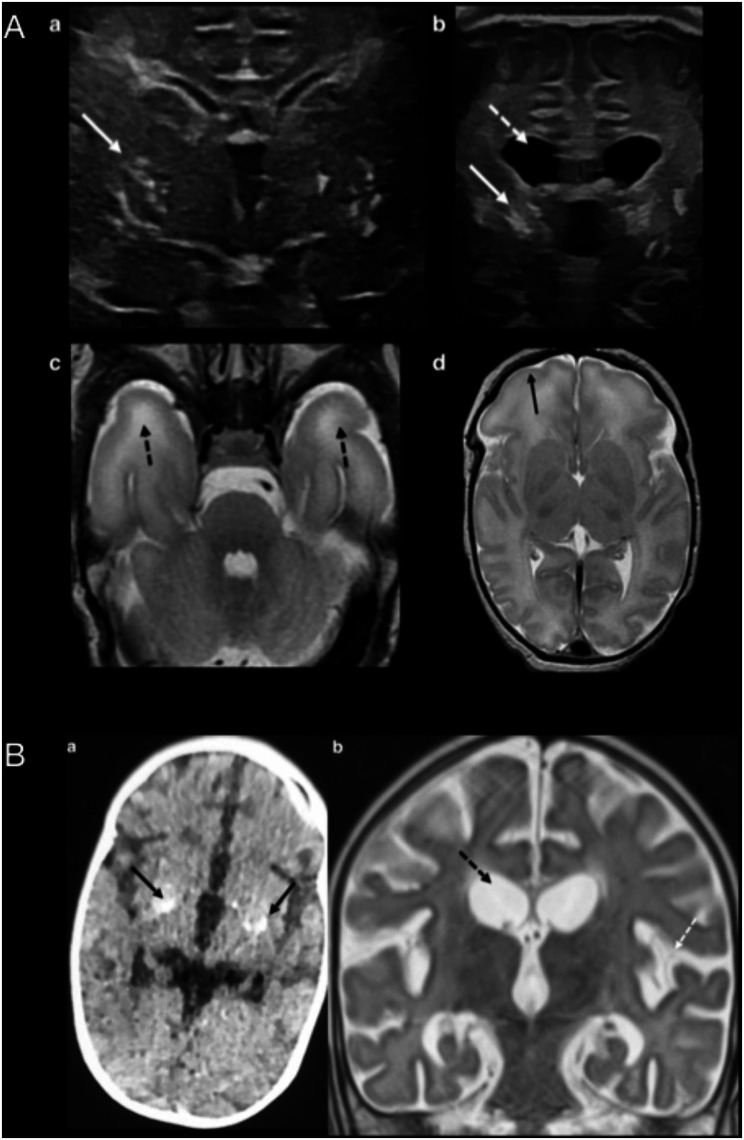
Neuroimaging findings. A Brain ultrasound (II:5) at birth (a) and at 3 months (b). Brain MRI T2-weighted axial images at 10 days (c and d). In (a) bilateral thalamostriate vasculopathy (white arrow) is shown. At 3 months (b) the extent of vasculopathy had increased (white arrow) and ventriculomegaly had developed (dashed white arrow). MRI (c) identified bilateral anterior temporal lobe white matter hyperintensity (dashed black arrows) and (d) simplified frontal lobe gyration pattern (black arrow). B Brain CT axial image (a) and coronal brain MRI T2-weighted (b) at 6-month-old (II:4). In (a) bilateral basal ganglia calcification is noticed (black arrows). In MRI (b) ventriculomegaly (dashed black arrow) and increased subarachnoid space (dashed white arrow) is remarkable
